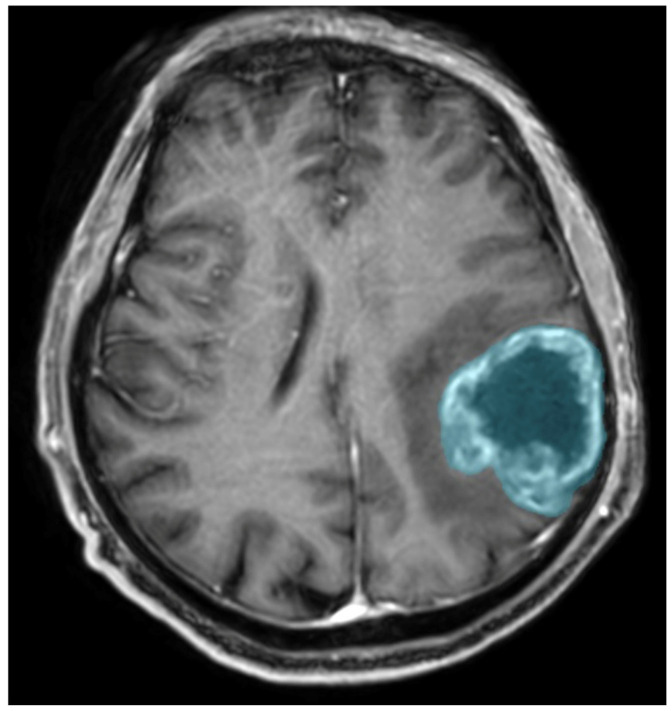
Glioma CNS WHO 4 in the left parietal lobe. T1-weighted image after administration of contrast agent; the blue color was used to mark the tumor segmented by the semi-automated method.
